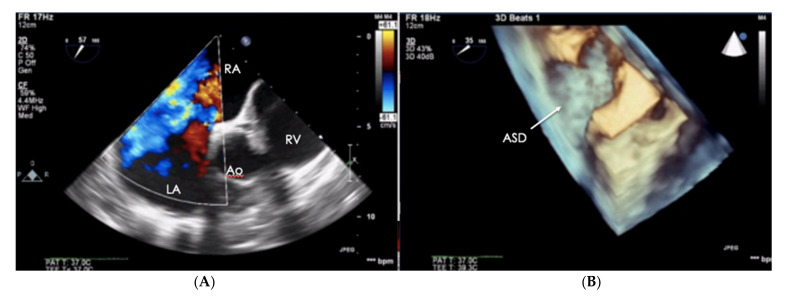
Patient with PAH-CHD–atrial septal defect. (A) 2D-TTE visualization, short-axis view (SAX). (B) The same defect visualized in the transthoracic echo (TTE), 3D visualization. PAH-CHD: pulmonary arterial hypertension-congenital heart disease; 2D-TTE: two-dimensional transthoracic echocardiography.
